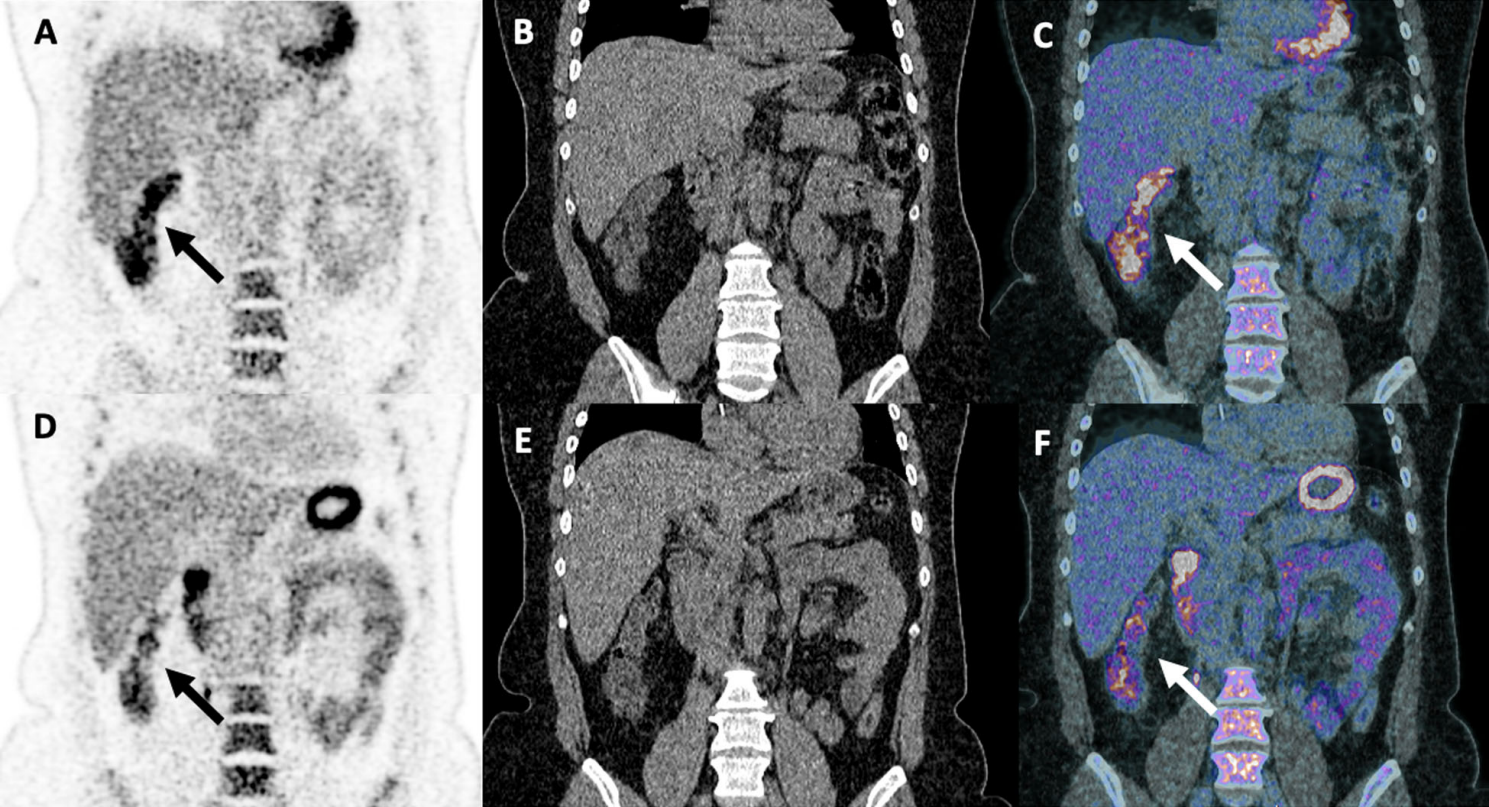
Partial pathological uptake of the right colon suspecting early signs of colitis in a 48-year-old woman with stage IV melanoma treated with two cycles of ipilimumab (anti-CTLA-4) and nivolumab (anti-PD-1). Treatment was discontinued due to grade II colitis diagnosed 3 weeks after the first ^18^F-FDG PET/CT scan [arrows; coronal PET (A), CT (B) and merged PET/CT (C) images], which required high-dose steroid treatment for 3 months. A decreased right colon uptake was observed on follow-up ^18^F-FDG PET/CT examination performed 4 weeks after the introduction of steroid treatment [arrows; coronal PET (D), CT (E) and fused PET/CT images (F)].
